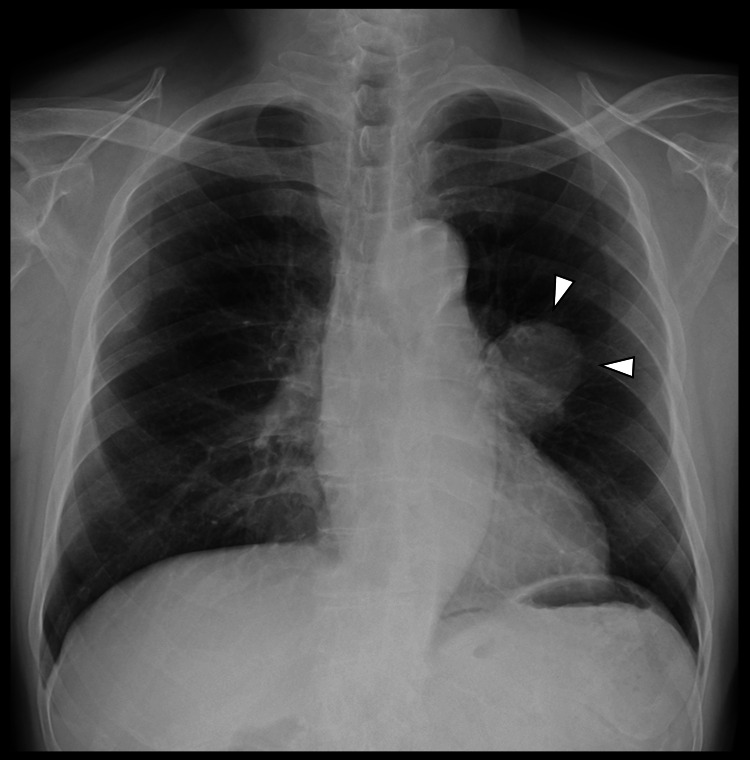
Preoperative chest radiograph. A mass is identified in the left lung (white arrowheads).
